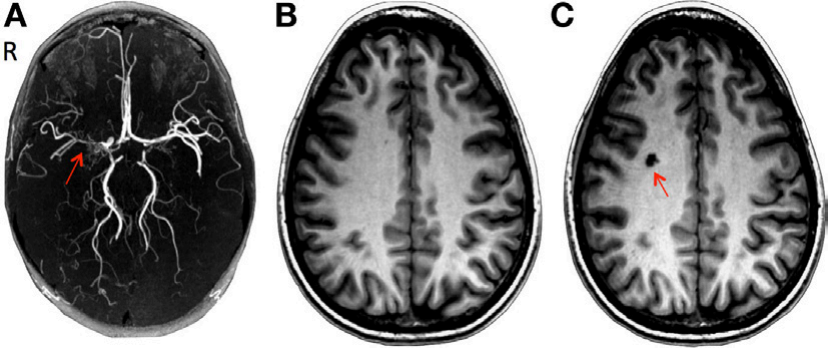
(A) Three-dimensional time-of-flight magnetic resonance angiography (MRA): severe stenosis in the right middle cerebral artery (red arrow). (B) Brain T1-weighted 3D spoiled gradient echo sequence MRI in June 2007 after initial presentation and (C) after the development of a lacunar infarct in the right centrum semiovale (red arrow) during a follow-up in September 2007.
